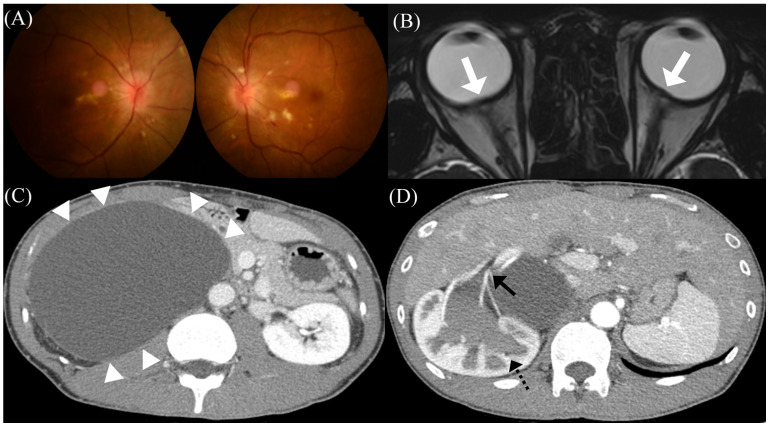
Initial clinical and radiological presentations of the patient. (A) Initial fundus photography showing bilateral papilledema and visible hard exudates at the posterior pole. (B) T2-weighted brain magnetic resonance images showing the flattening of the posterior sclera bilaterally, suggestive of intracranial hypertension (white arrows). (C) Abdominal computed tomography with contrast enhancement showing a huge benign cystic lesion (white arrowheads), (D) resulting in hydronephrosis (black dotted arrow) and (D) extrinsic compression of the renal artery of the right kidney (black arrow).
